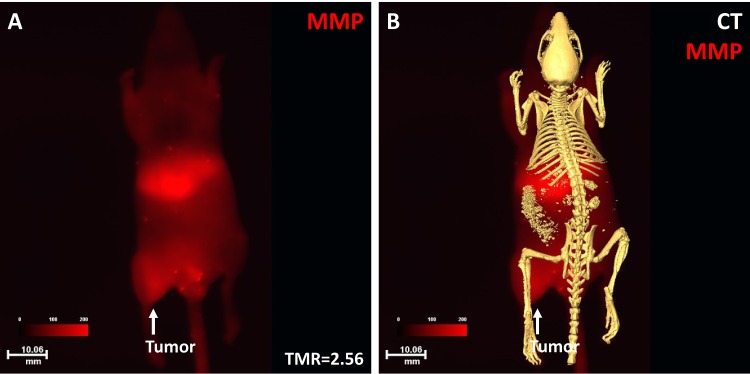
Optical and CT imaging of MMP binding to the NPC tumor Optical imaging showed that the NPC tumor had high signal intensity with a TMR of 2.56 (A). The merged images showed the anatomic location of the tumor and MMP signal distribution at the whole-body level (B).
